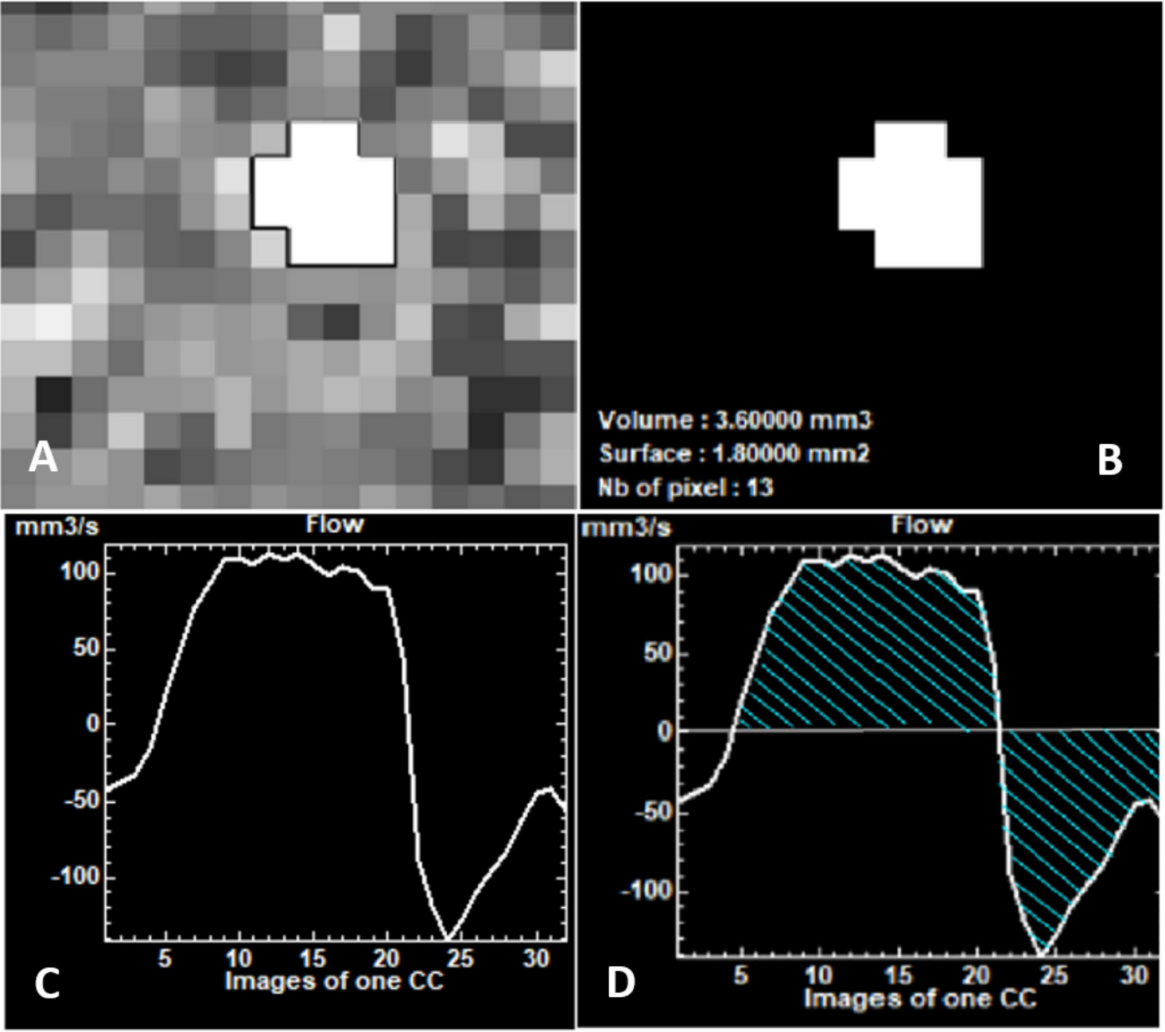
A) Phase-contrast MRI (PC-MRI) data of the aqueduct of Sylvius. B) Semi-automatic segmentation of the aqueduct of Sylvius using Flow software (developed in-house at Amiens-Picardie University Hospital) to isolate the region of interest for measuring the cerebrospinal fluid (CSF) stroke volume (SV) passing through the aqueduct during the cardiac cycle. C) CSF flow curve through the aqueduct plane over the cardiac cycle, generated using Flow software and the semi-automatic segmentation described above. D) Integration of the CSF flow curve, yielding the stroke volume of CSF passing through the aqueduct of Sylvius during the cardiac cycle
